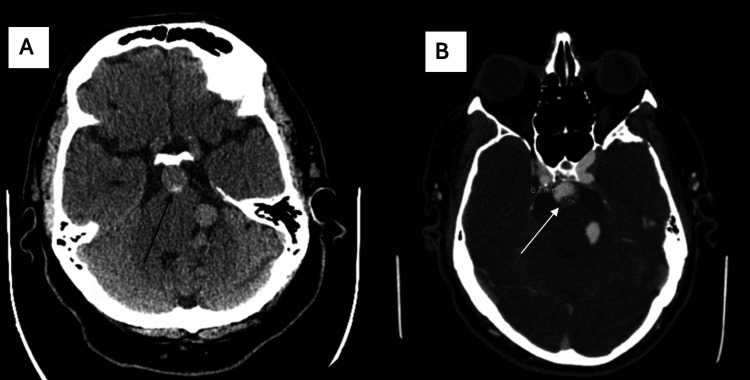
A. CT head without contrast, dolichoectatic vertebral artery. B. CTA head shows vertebrobasilar dolichoectasia noted with a large, long filling defect (thrombus) seen involving the mid basilar artery.
